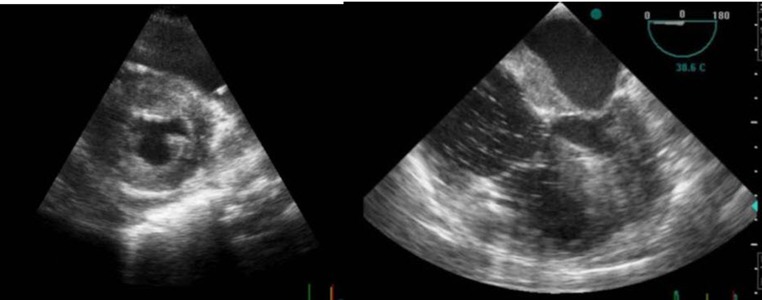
Transthoracic and transesophageal echocardiography showed interatrial and left ventricular thickening
